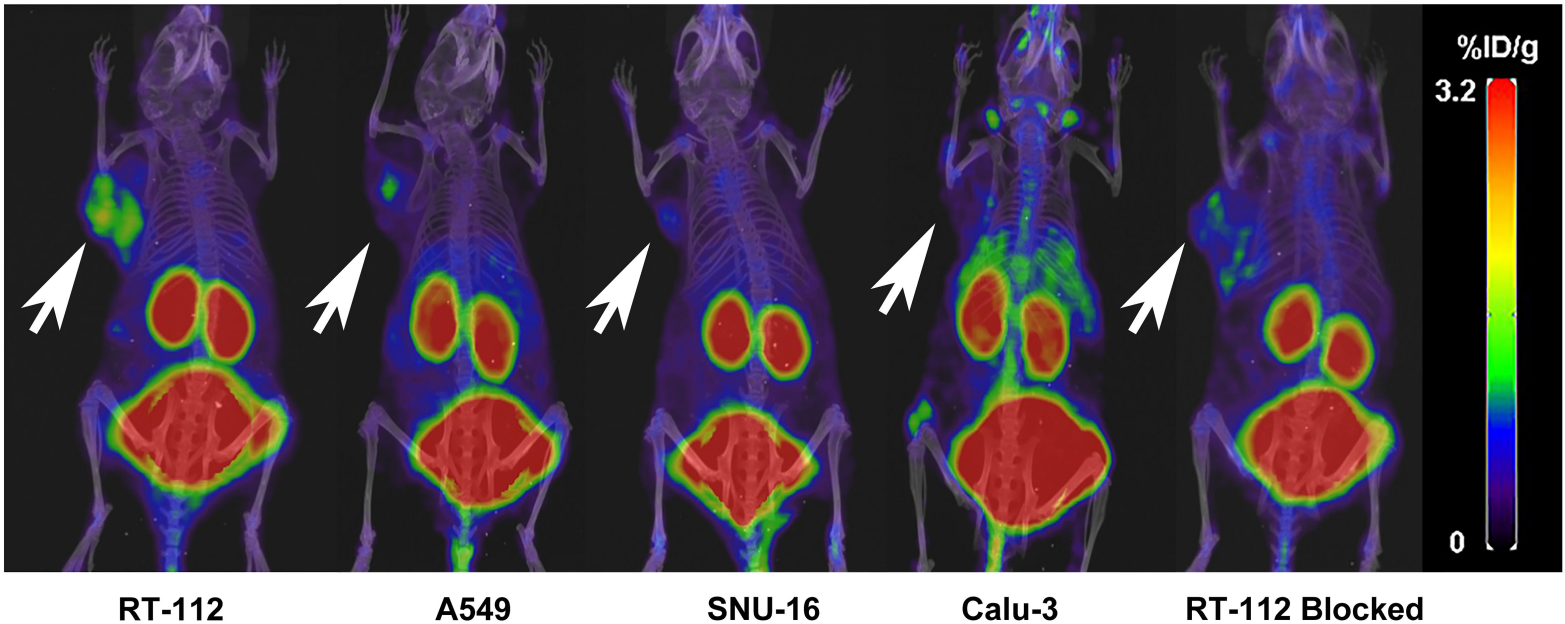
Comparing RT-112 (FGFR1-high expression) xenografts, the other xenografts showed lower tumor uptake 30 min post-injection (3D MIP of micro-PET/CT imaging). Arrows indicate tumors.
